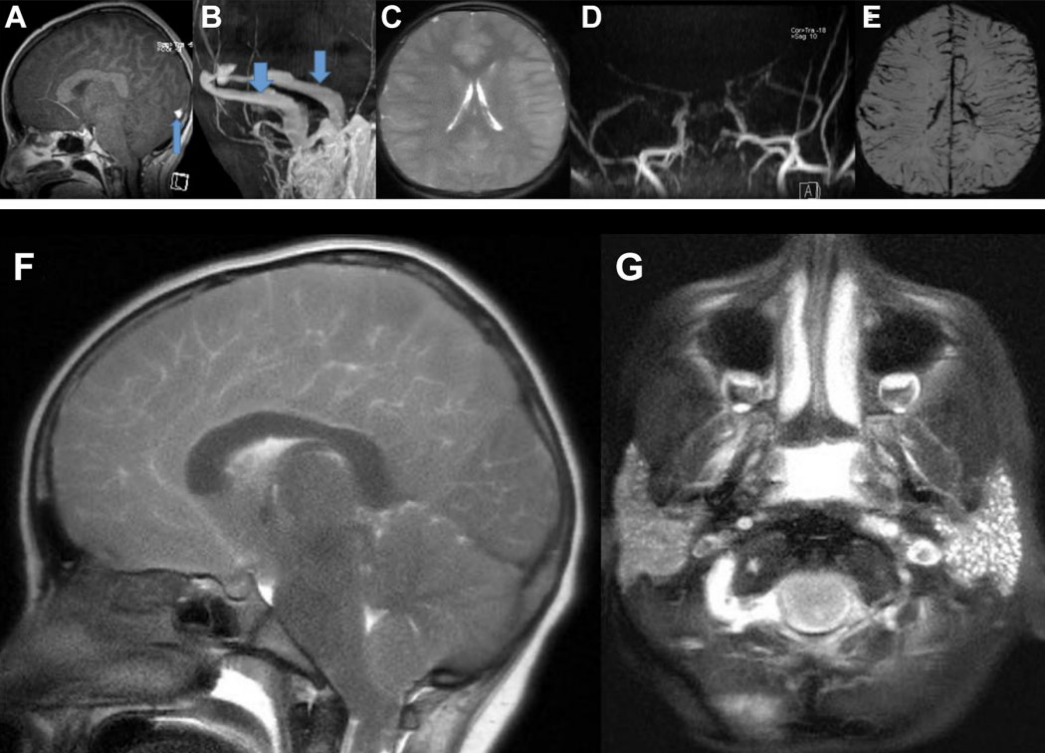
Brain imaging (MRI/MRA/MRV). day #1 brain MRI (A) sagittal T1 image demonstrates downward herniation with decreased size of the suprasellar cistern, posterior fossa crowding causing brainstem compression against the clivus and developing tonsillar herniation. (B) MRV demonstrates patent jugular veins and transverse sinuses without thrombosis. The sagittal sinus and deep venous system are not visible. (C) T2 axial images reveal diffuse cortical swelling mild, global gray matter hyperintensity, and slit-like ventricles suggesting brain edema. (D) coronal MRA image demonstrates near complete or complete loss ACA, MCA, PCA and basilar flow. (E) susceptibility-weighted imaging shows exaggerated cortical veins consistent with engorgement vs thrombosis. the subsequent MRI sagittal T1 (F), day #2 demonstrates tonsillar herniation down to the level of C2. MRIs on day #1 and 2 revealed bilateral parotid cysts (G), suggesting parotitis, which has been reported in cases of COVID-19 infection.^
